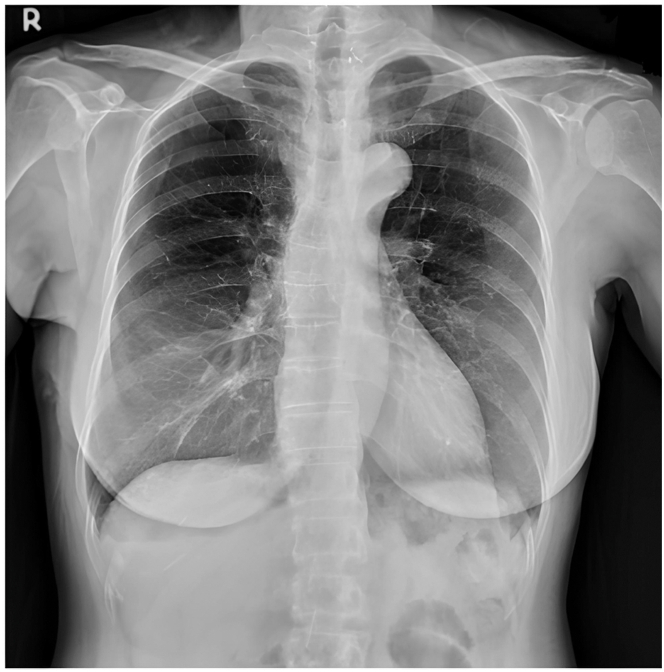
Postoperative chest roentgenogram (posteroanterior view) demonstrating no abnormalities in the right (R) thoracic cavity, with good lung expansion.
